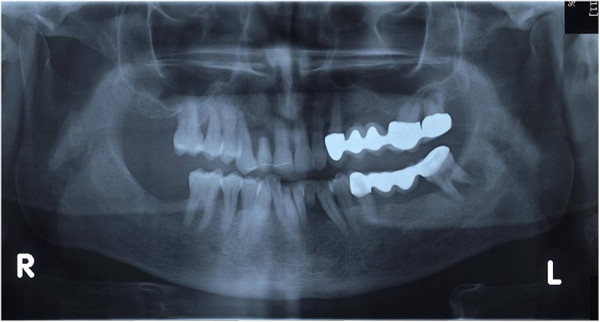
Panoramic X-ray showing vertical bone loss.
